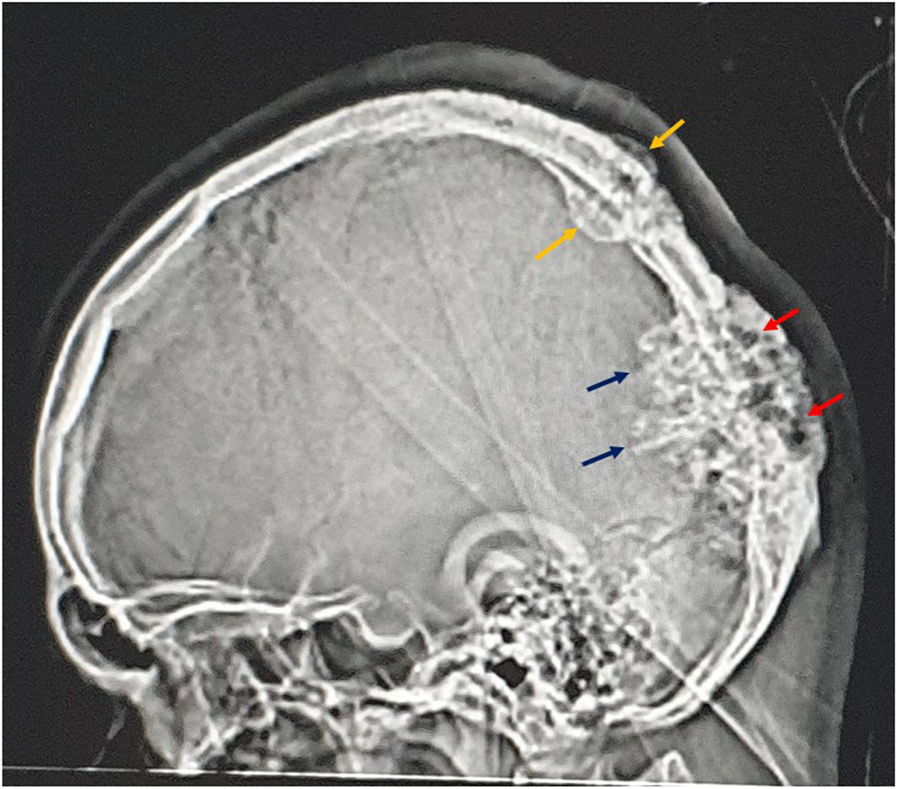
Scout view of preoperative CT scan showing parietal (yellow arrow) and occipital bony lesions. The occipital lesion was larger with internal trabeculations (red arrows), and a major intracranial part with spiculated margins (blue arrows)
